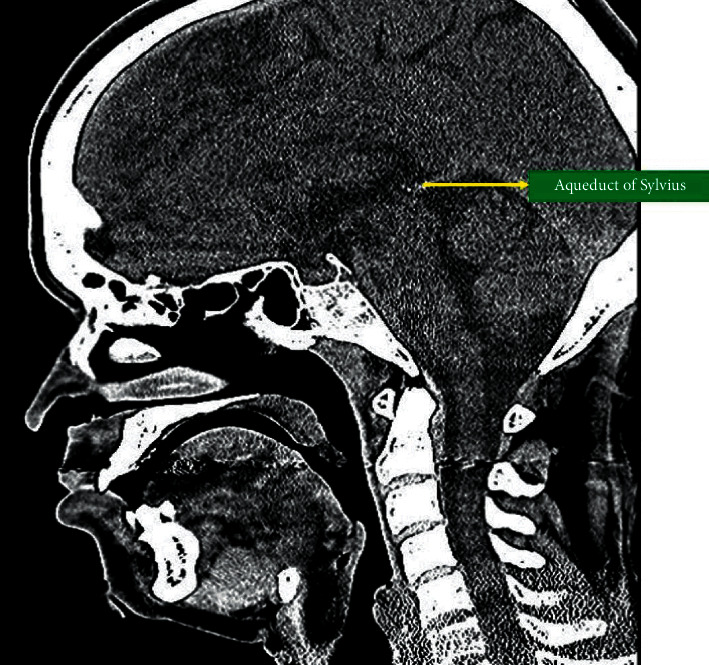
The aqueduct of Sylvius in the 2D midsagittal CT image.
